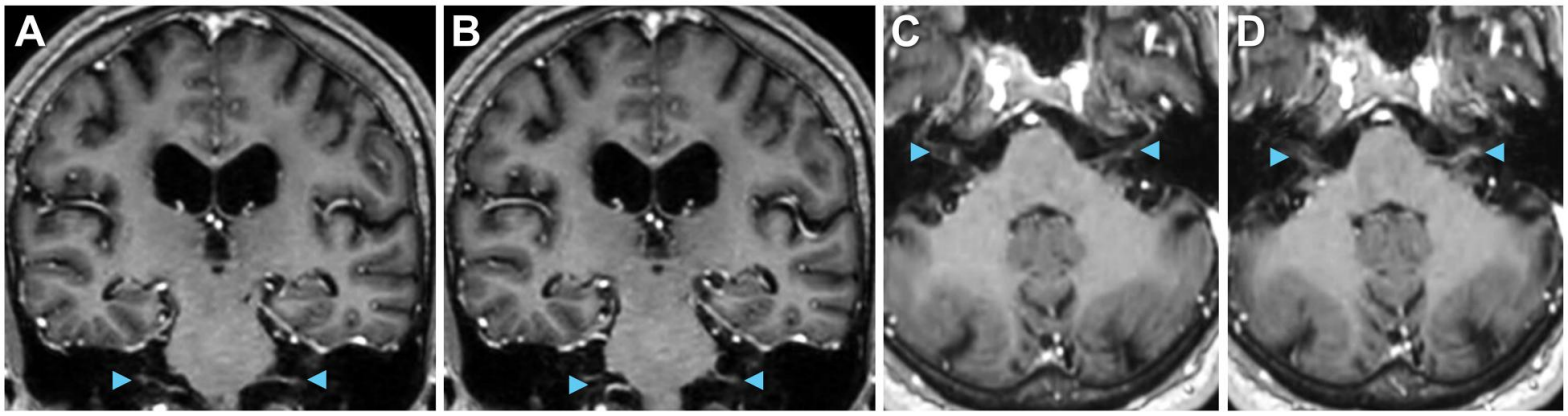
Cranial nerves on MRI. Suggestion of cranial nerve involvement (arrowheads), including cranial nerve V on (A and B) coronal and (C and D) axial T1 post-contrast MRI.
